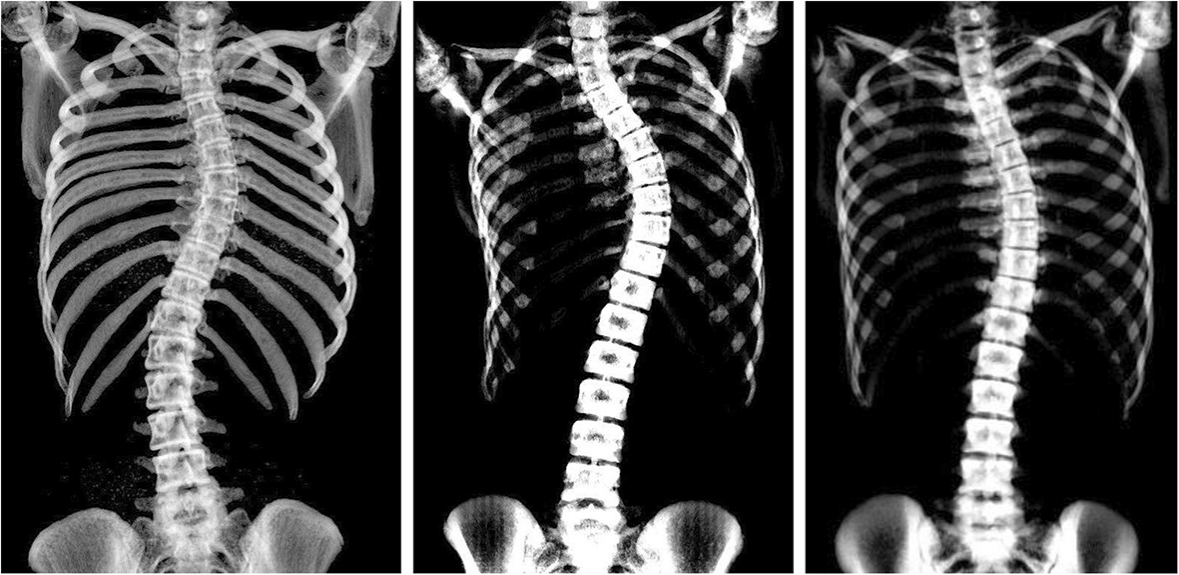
Reformatted coronal CT images of idiopathic scoliosis patients, used for supine Cobb angle measurements.
